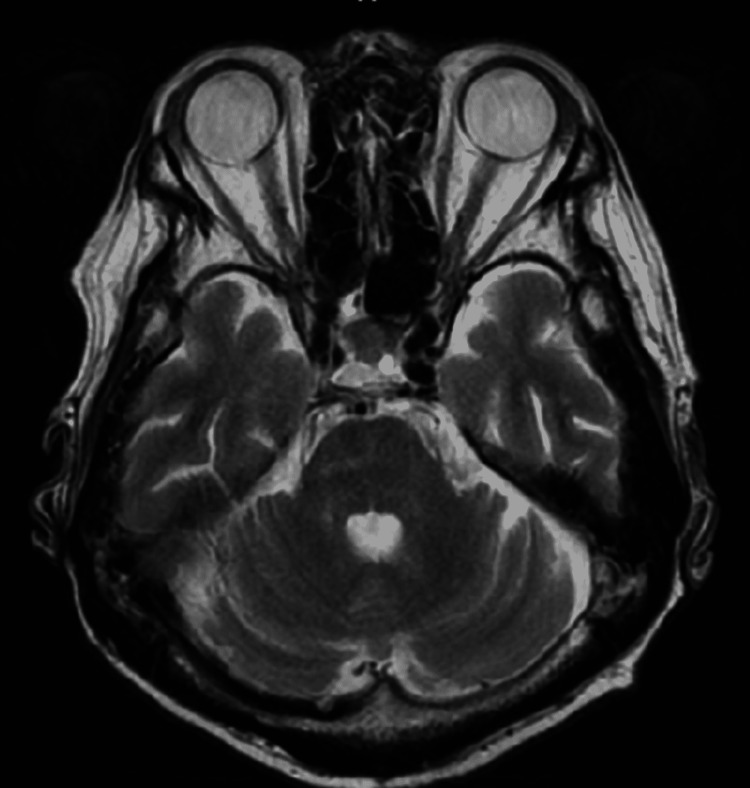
T2-weighted MRI of the head shows no abnormalities, including the optic nerve.
